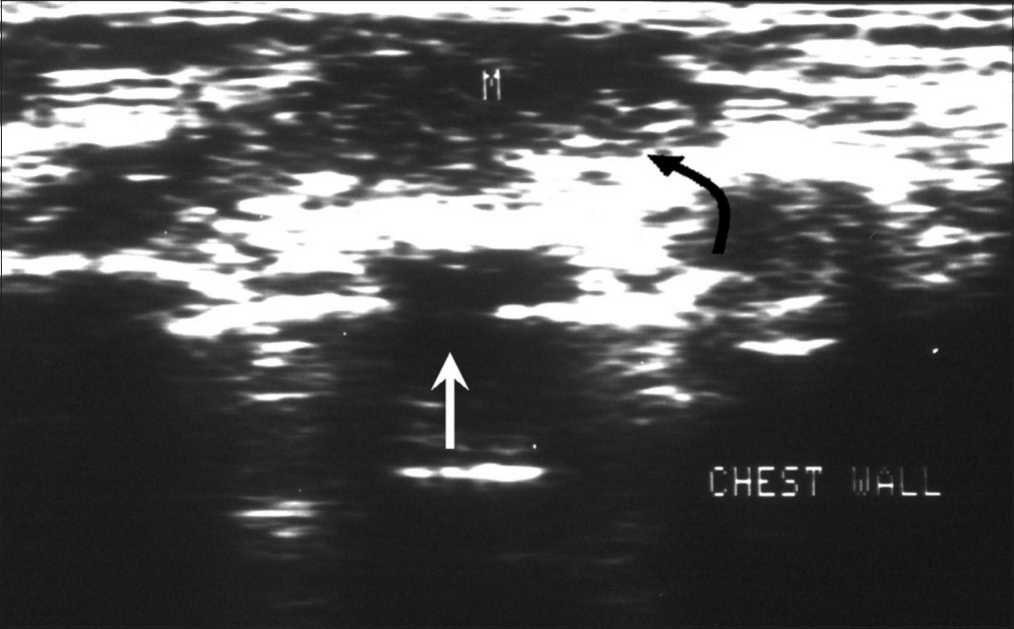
Rib tuberculosis – patient 6. Ultrasonography of the chest wall shows rib destruction (white arrow) with an associated hypoechoic abscess (“M,” curved black arrow)
